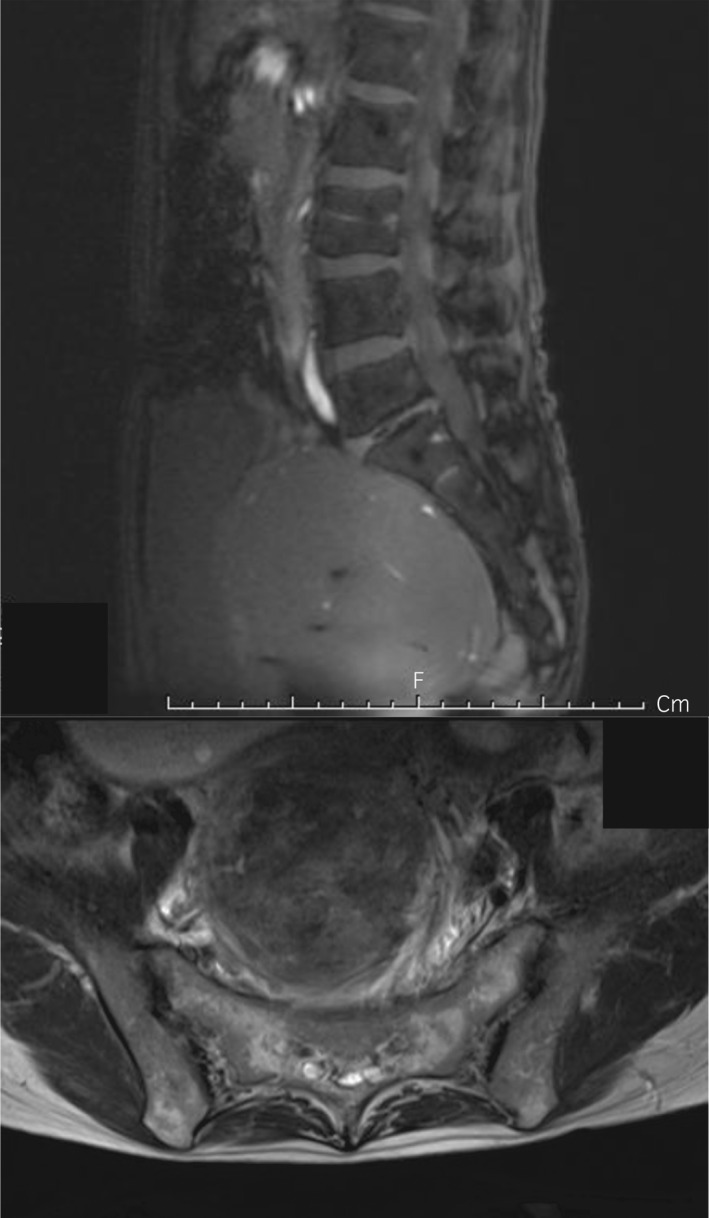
Abdominal MRI of case 2. An MRI scan revealed a UL 10 cm in diameter. Although the pelvic plexus itself could not be visualized, the size and the posterior‐base location of the tumor was thought to compress the pelvic plexus in this patient.
